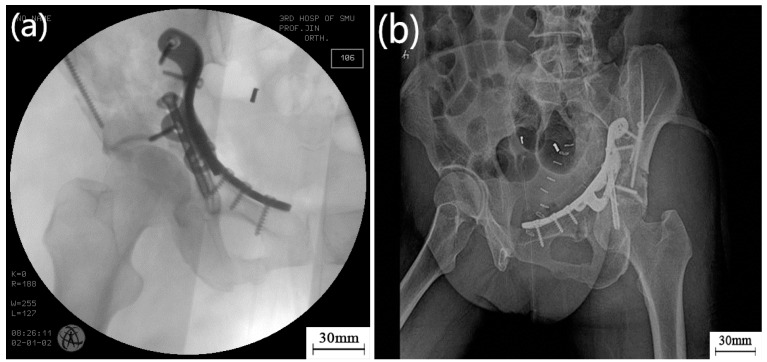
Examination of fixation effect of the bone plate during and after the operation: (a) C-arm image during operation; and (b) X-ray image following surgery.
